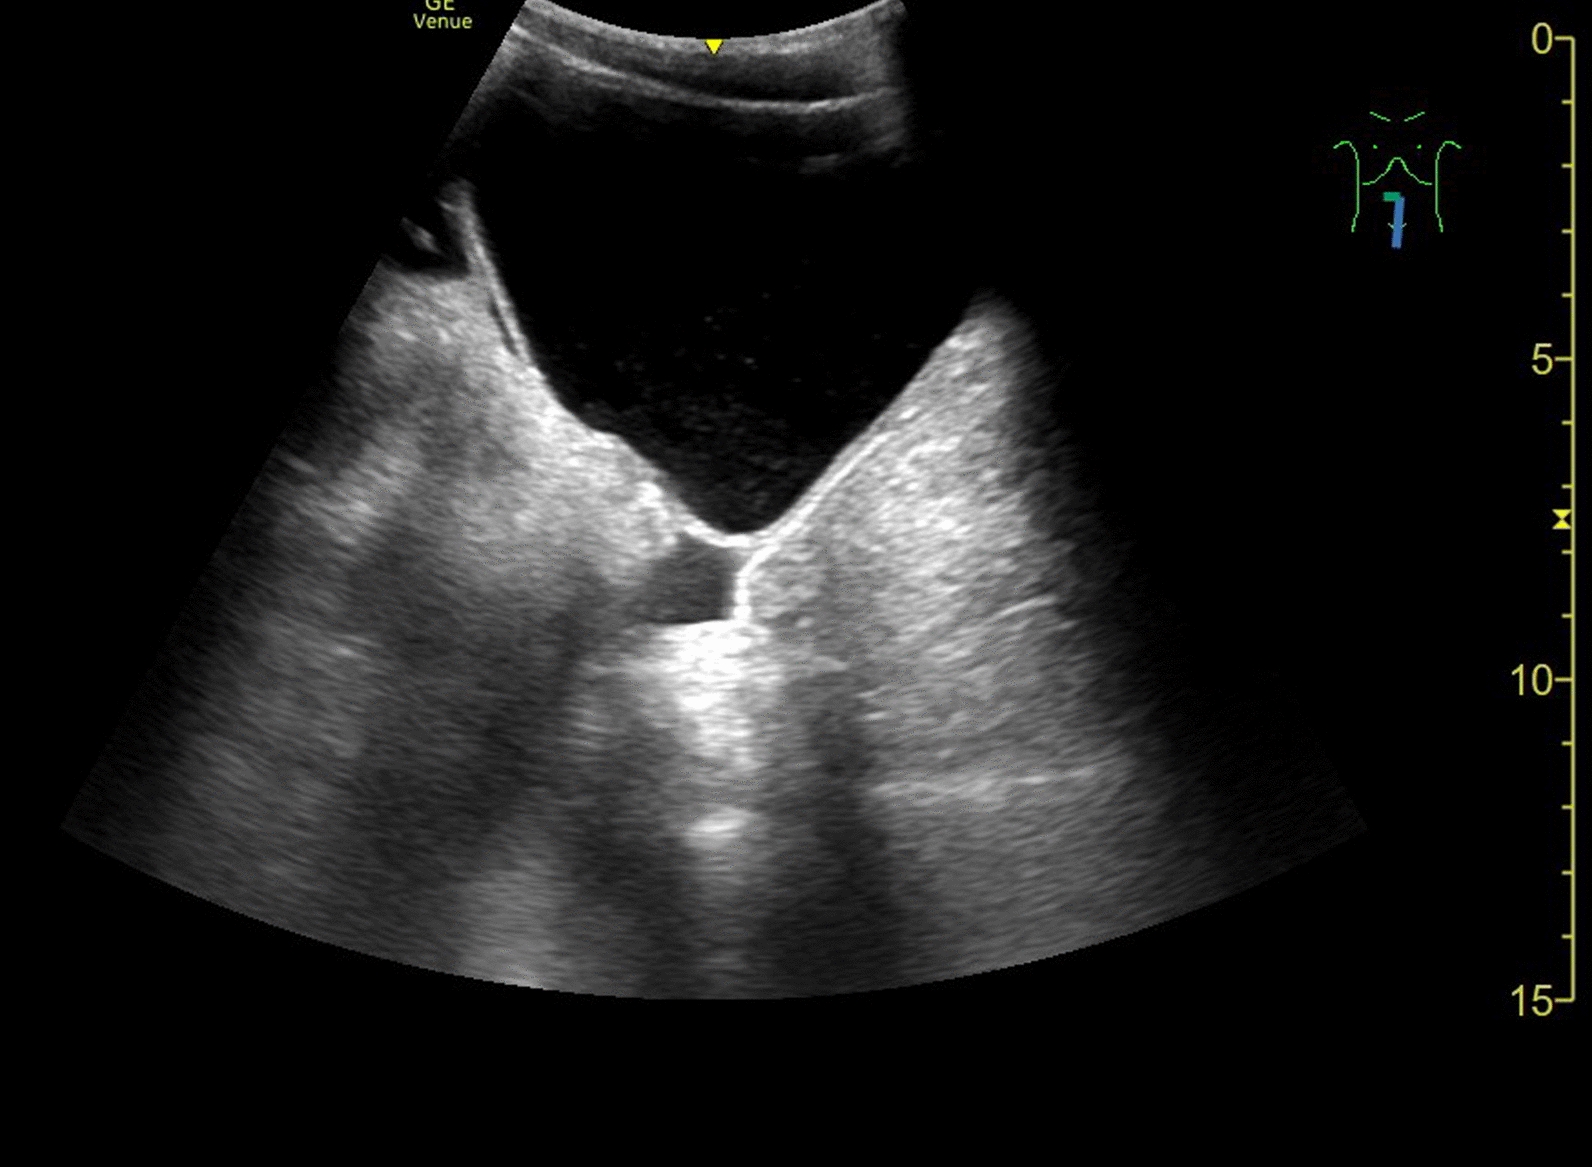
Free fluid in the lesser (true) pelvis as seen on transabdominal ultrasound
